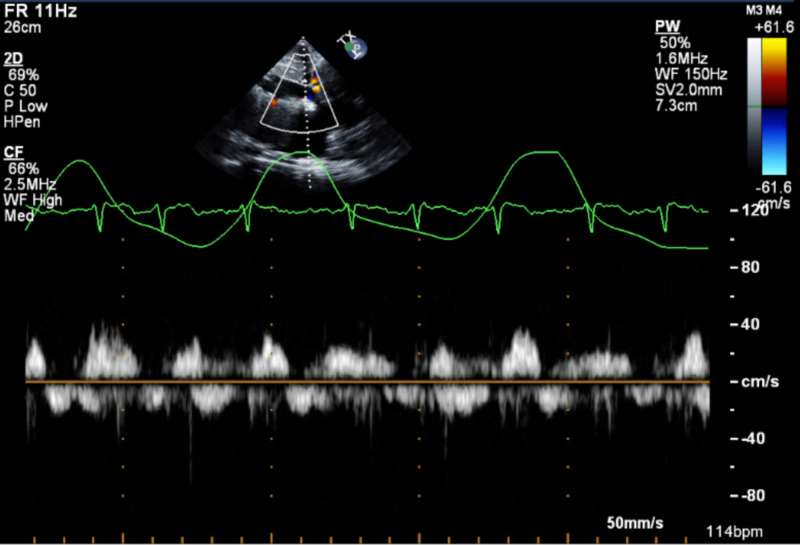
Pulsed wave Doppler echocardiogram at admission showing the blunted respirophasic motion of inferior vena cava
